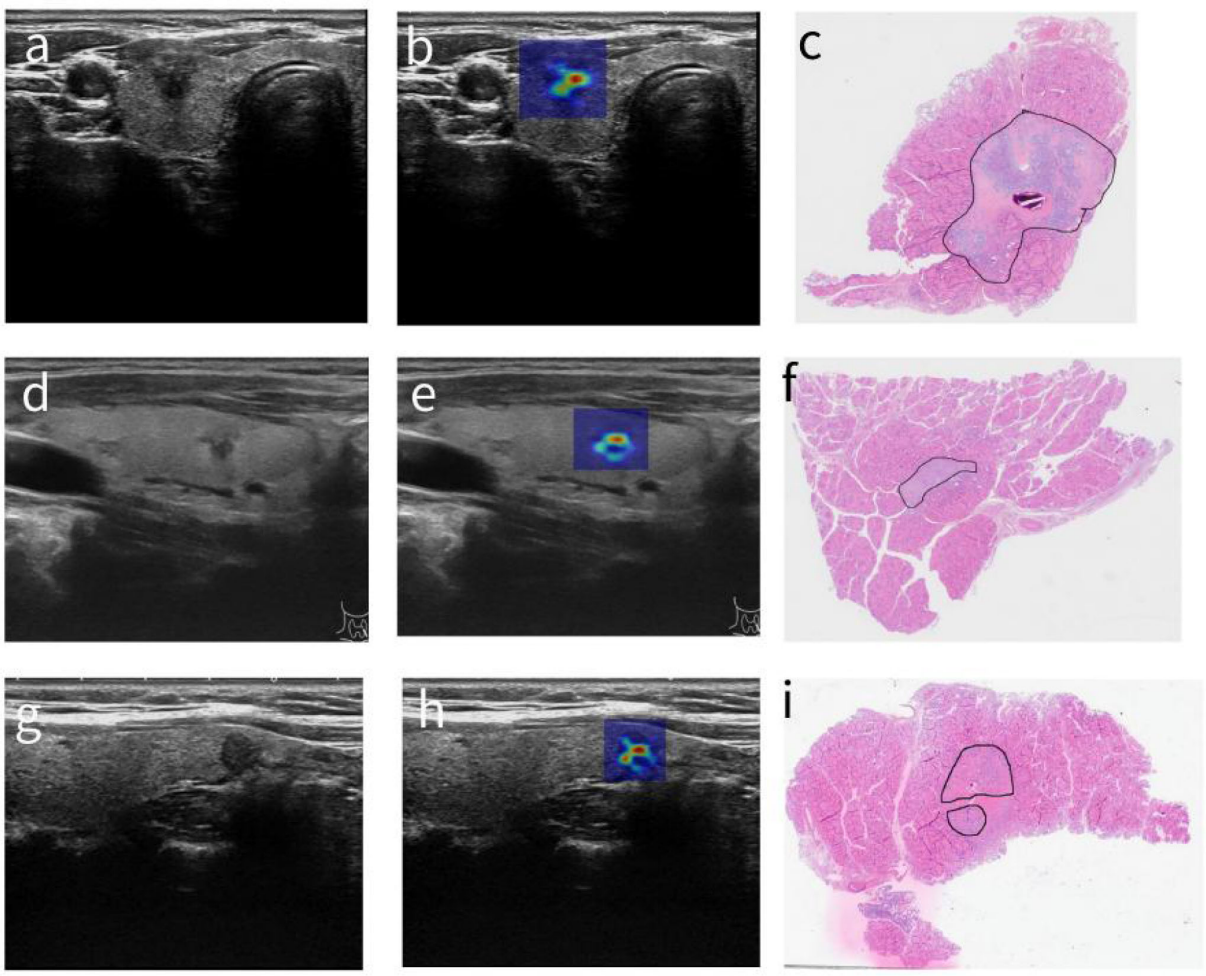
Correspondence of post-operation pathological images and malignancy heat maps computed on ultrasound images. (A, D, G) The original thyroid ultrasound images. (B, E, H) The superimposed images for the corresponding nodules and heat maps. (C, F, I) The cropped zoomed-in pathological images.
